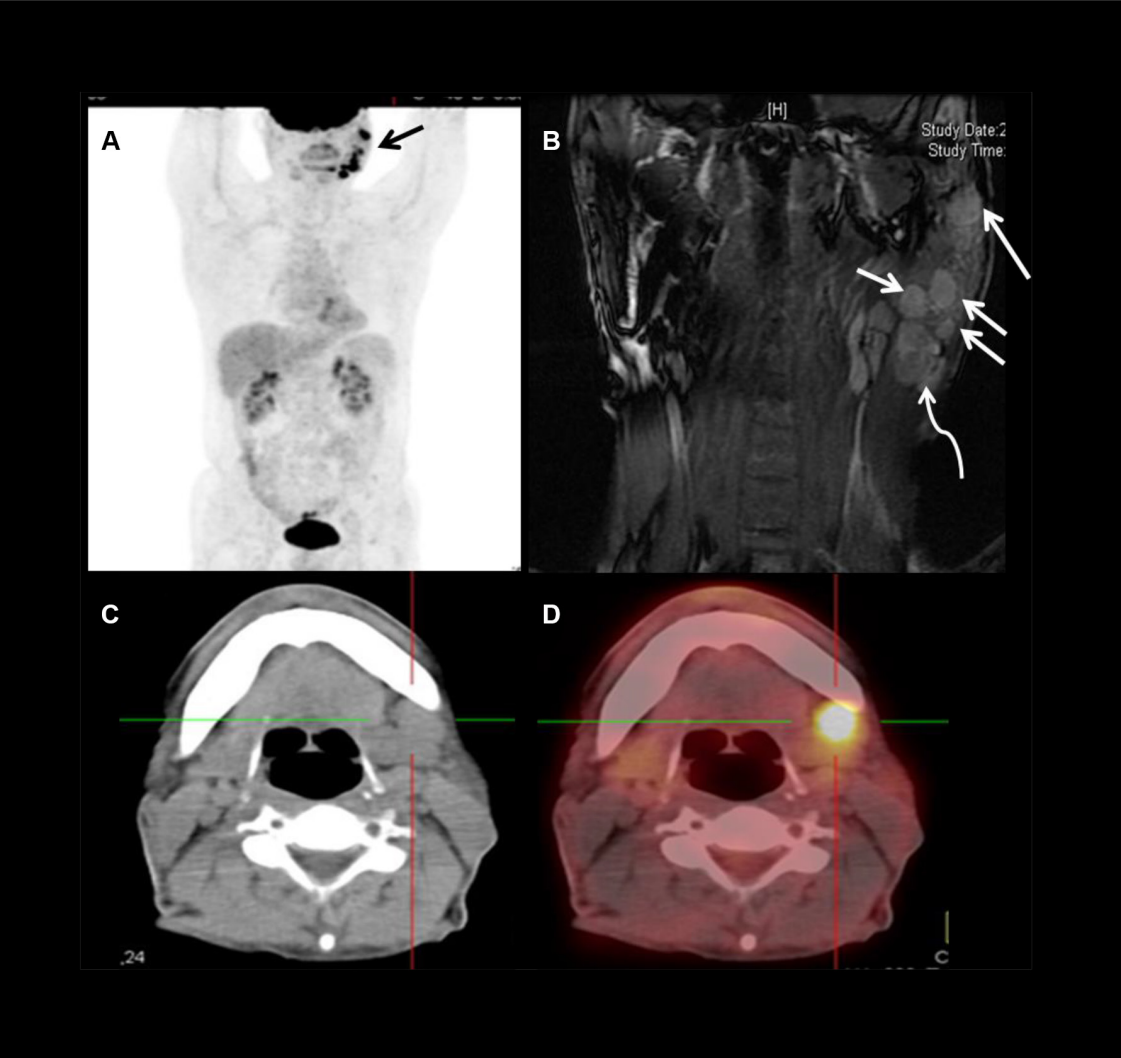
Images showing level Ib and level VIII nodes metastases in a 58 years old male patient with LELC of the left parotid gland (A) PET panorama demonstrated multiple nodules (arrow) in the left parotid and upper neck with high uptake of FDG. (B) Coronal MRI showed multiple metastases (arrows) in and just beneath (curved arrow) the left parotid. (C and D) CT-PET suggested level Ib nodal involvement.
